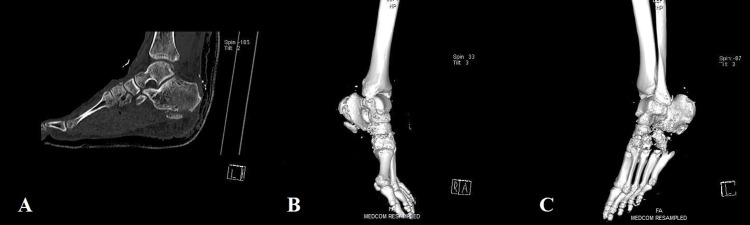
CT of the foot and ankle (sagittal view [A] and reconstructed images [B, C]) showing the osseous lesions.
